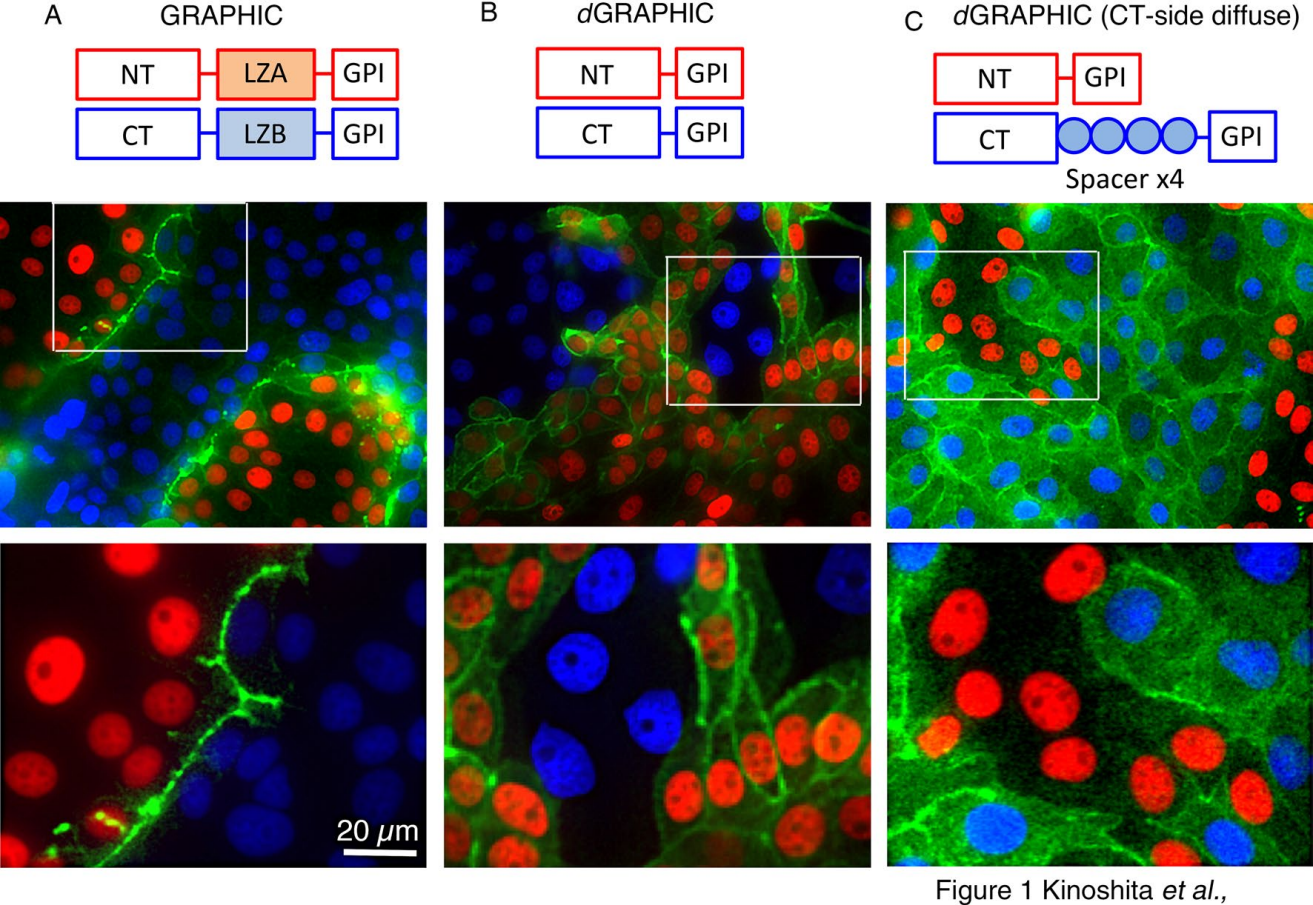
Distribution of reconstituted GFP is dependent on GRAPHIC probe molecular structure. A red nucleus (labeled by H2B-mCherry) indicates an NT cell, and a blue nucleus (labeled by H2B-Azurite) indicates a CT cell. (A) GRAPHIC: NT probe containing an acidic leucine zipper domain (LZA) and CT probe containing a basic leucine zipper domain (LZB). Reconstituted GFP was observed only at the boundary between NT and CT cells. White box indicates position of higher magnification view. (B) *d*GRAPHIC: Leucine zipper domain was removed from both NT and CT probes. *d*GRAPHIC showed almost all reconstituted GFP signals on NT cells, compared with CT cells. (C) A probe pair consisting of NT and CT probes with 3 spacer domains (15 aa × 3) inserted between the GFP-CT fragment and GPI anchor domain. Reconstituted GFP was equally observed on both NT and CT cells. (D) Combination of NT and CT probes with 4 spacer domains (15 aa × 4). Reconstituted GFP was detected predominantly on CT cells. Scale bar, 40 µm and for higher magnification 20 μm.
